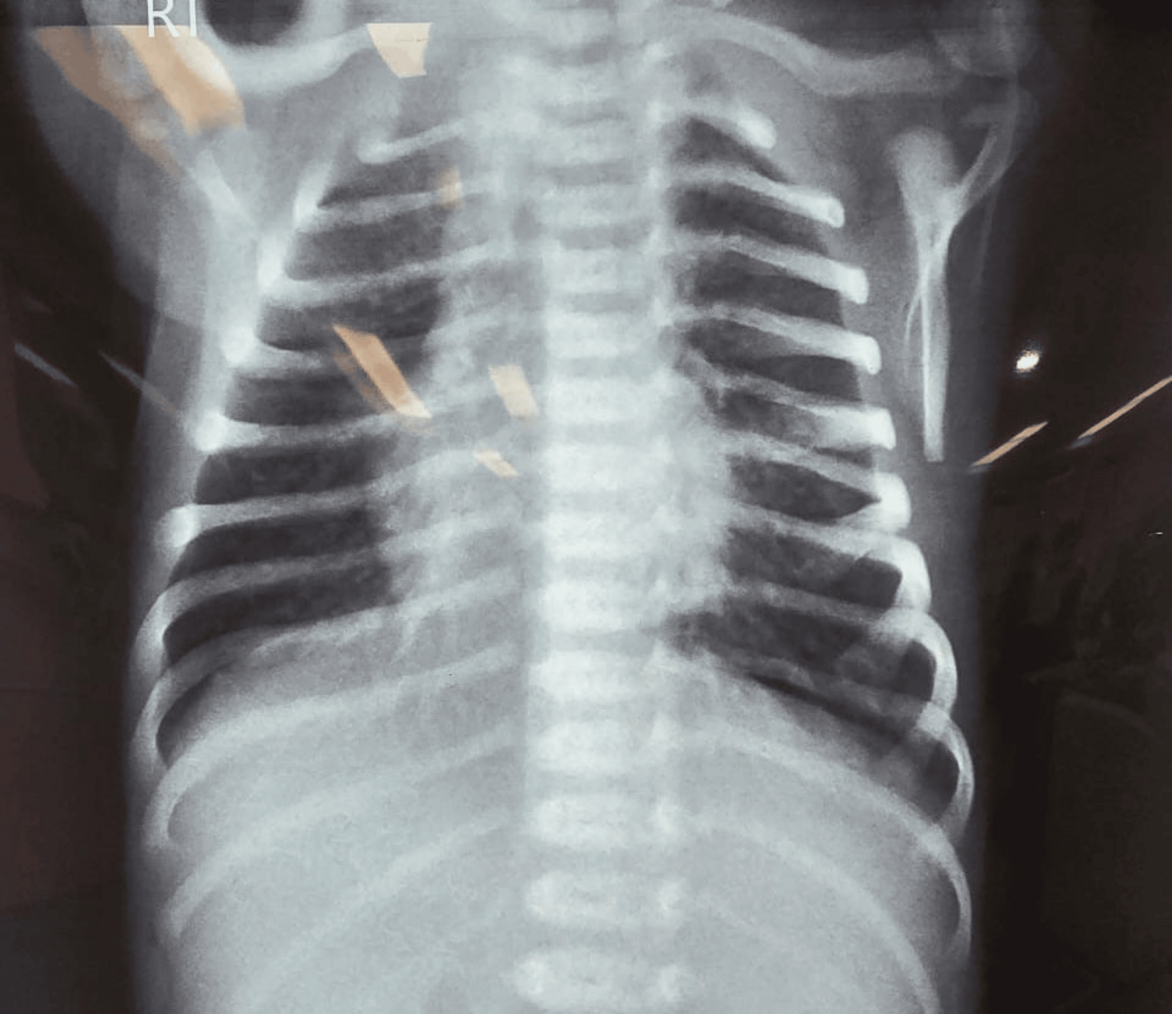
Day 05 chest X-ray of the patient showing resolution of previous lung infiltrates.
